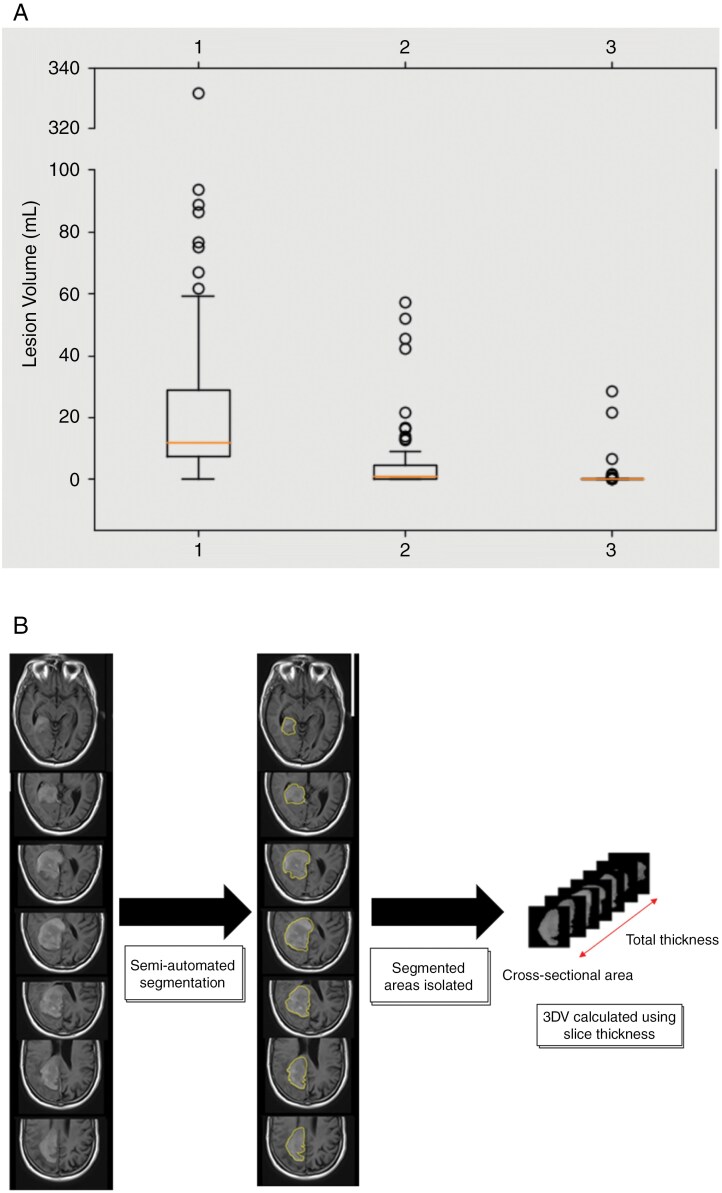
3-Dimentional Volume Data and Measurements. (A) Baseline (1), interim (2) and end of treatment (3) MRI volume data. (B) 3DV analysis.
